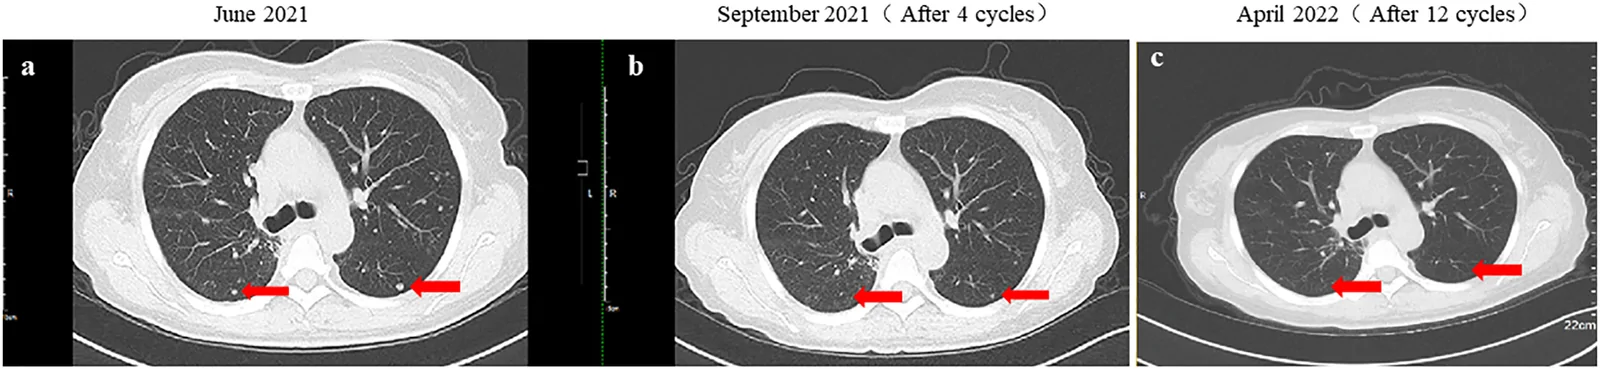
Chest CT scan showing (a) the baseline tumor lesion (arrows) before the 5^th^-line treatment with trastuzumab deruxtecan (T-DXd), (b) reductions in tumor lesion sizes (arrows) after 4 cycles of treatment with T-DXd, and (c) disappearance of tumor lesions after 12 cycles of treatment with T-DXd.
